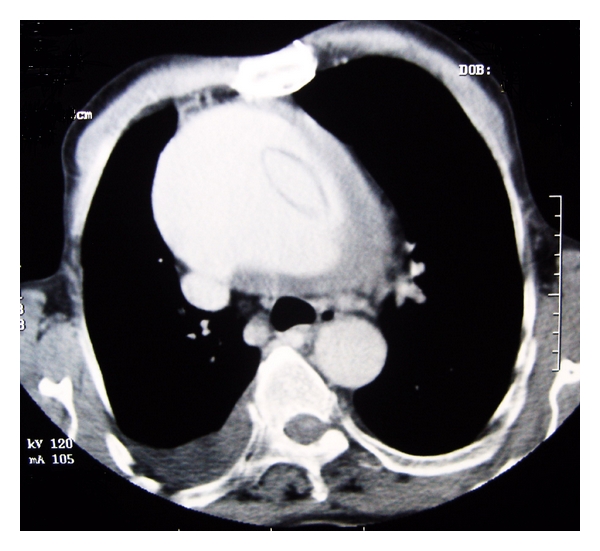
CT scan of the chest with an intimal flap separating the true and false lumen, mural thrombus near the intimal flap, aneurysm of the ascending thoracic aorta approximately 11 cm in diameter, and pleural effusion at right hemithorax.
